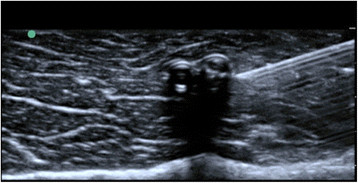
Ultrasound guided nerve block.
